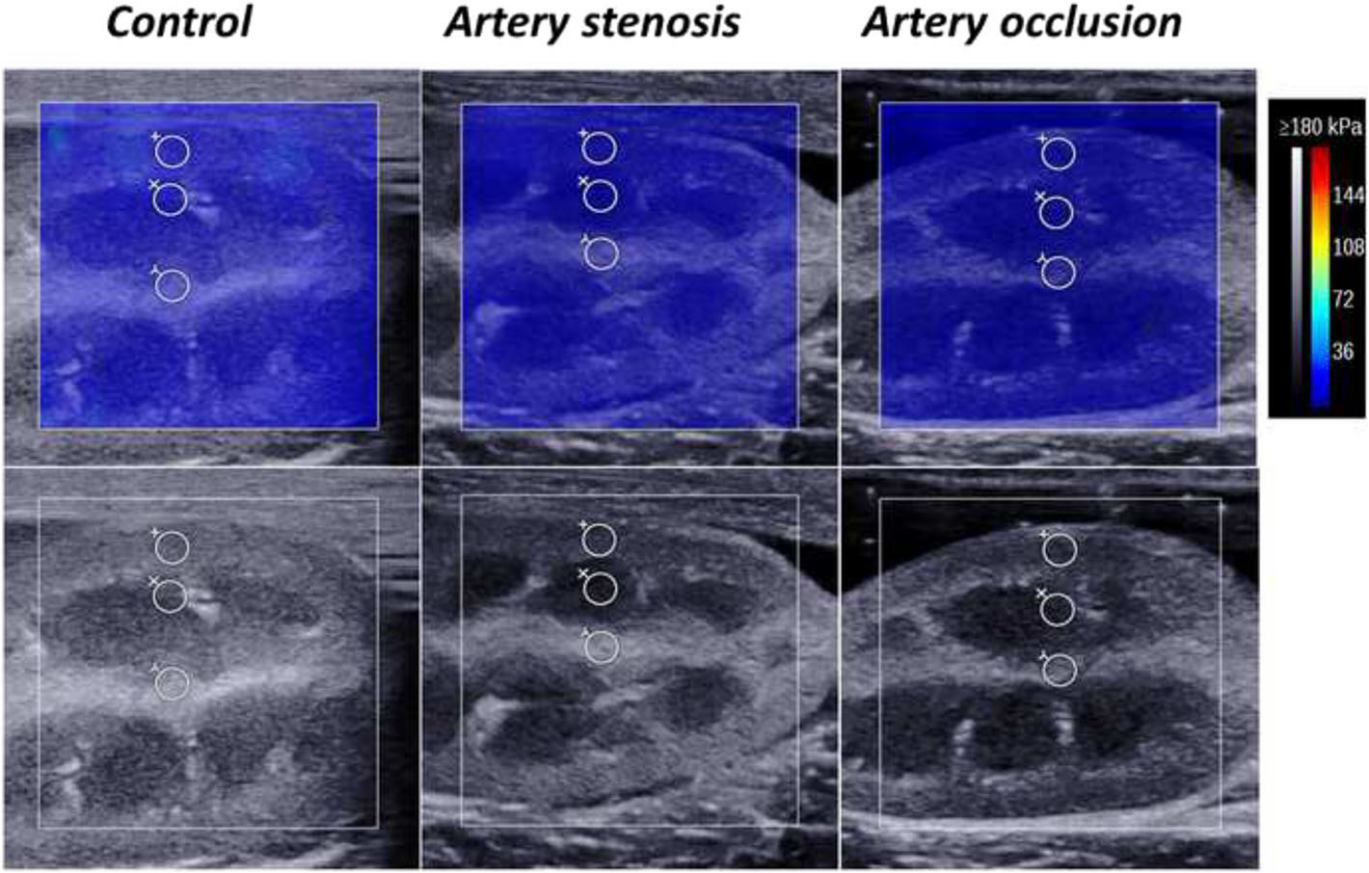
Representative images showing the progressive decrease in stiffness by SWE in one kidney with different degrees of stenosis of the renal artery. The lower and upper rows show the changes in ROI by grey-scale ultrasound and SWE, respectively. The colour map is the distribution of elasticity values scaled from 0 to 180 kPa
